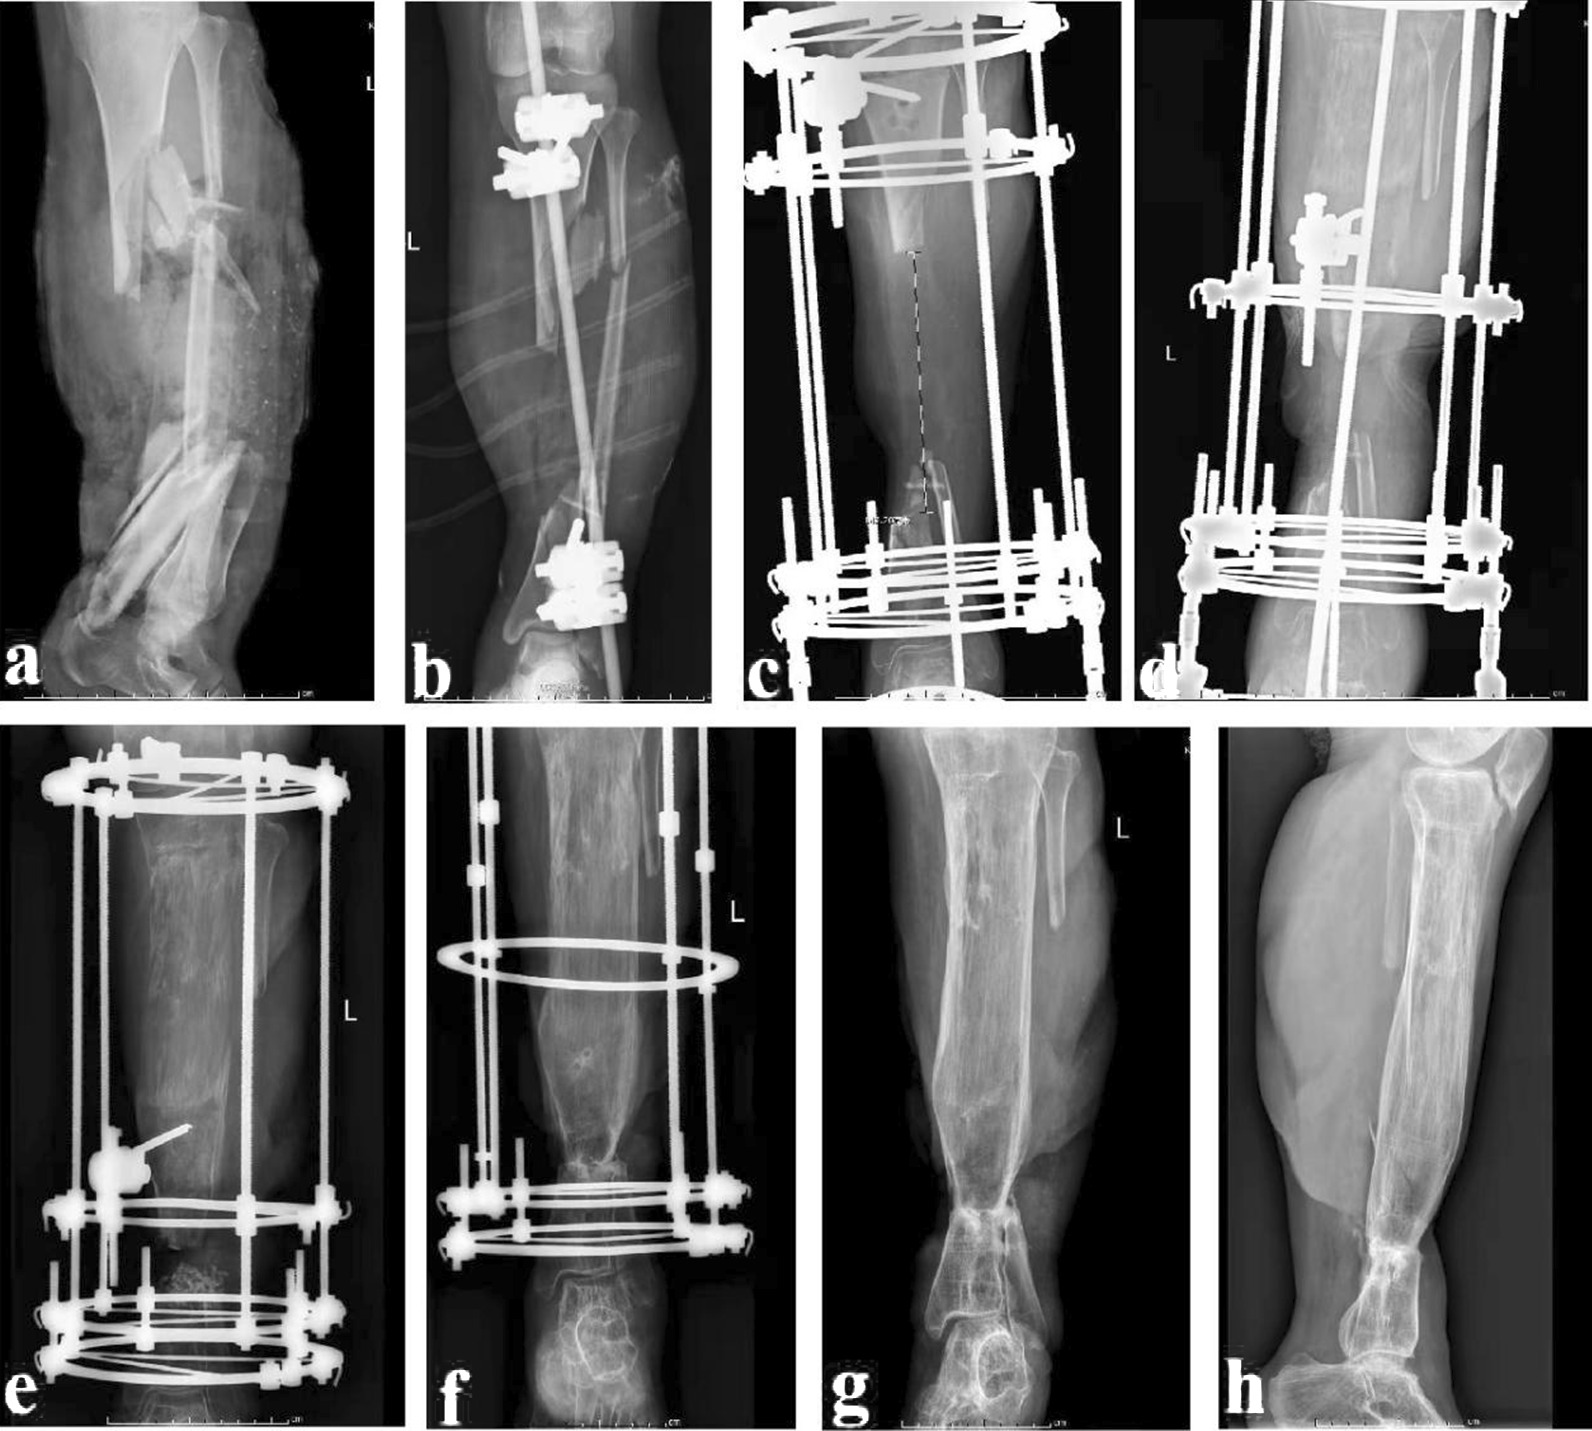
A 23-year-old female was diagnosed as severe tibial open fracture and treated with the TIBT technique. a X-rays after injury. b After debridement, X-rays of the affected tibia. c This patient was treated with the TIBT technique, 14 cm of tibial defects were observed. d–f The X-rays showed the bone transport process. g and h X-rays after removal of the Ilizarov external fixator. TIBT stands for traditional Ilizarov bone transport
